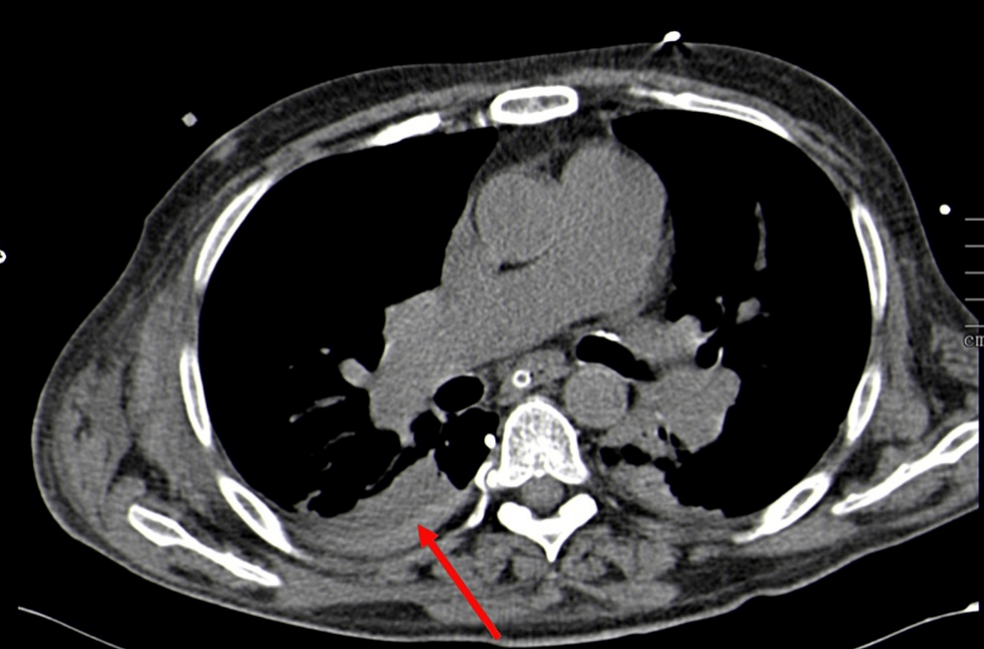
CTA scan showing hemothorax (red arrow) in the right pleural cavity (axial view) CTA, computed tomography angiography
